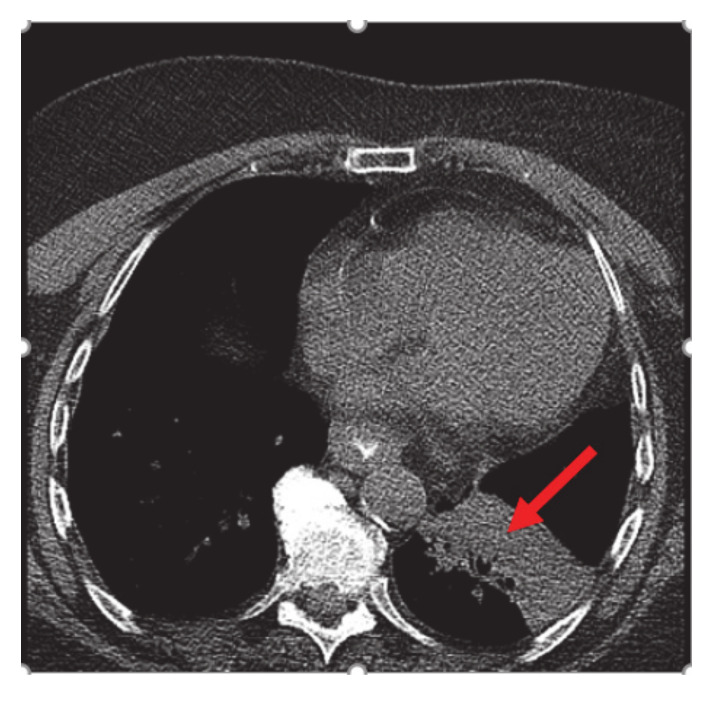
CT chest: left lower lobe consolidation/atelectasis (red arrow) with obscuration of proximal left lower lobe bronchus and mildly enlarged mediastinal nodes.
